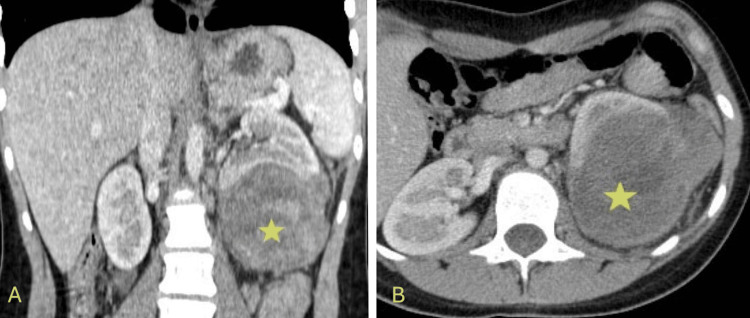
CT scan showing a left large renal mass that is occupying the mid and lower poles with heterogeneous enhancement (yellow stars). A: Coronal view of the CT scan; B: Axial view of the CT scan
